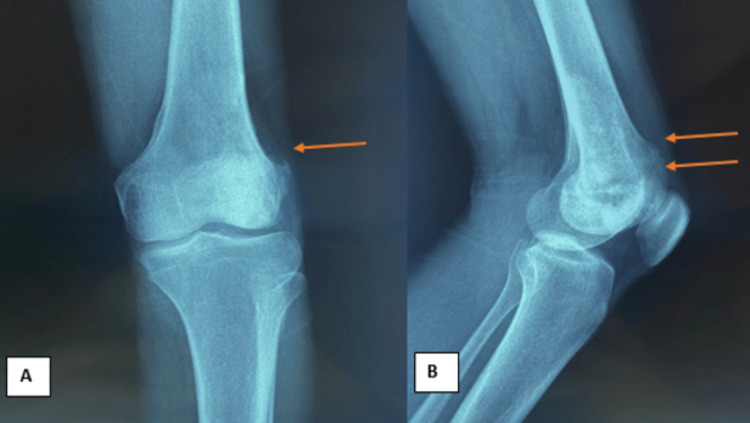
X-rays of the left knee during the initial presentation. (A) AP view reveals an osteoblastic epiphyseal-metaphyseal lesion. (B) Lateral view reveals the extension of the osteoblastic lesion to the entire external condyle.
